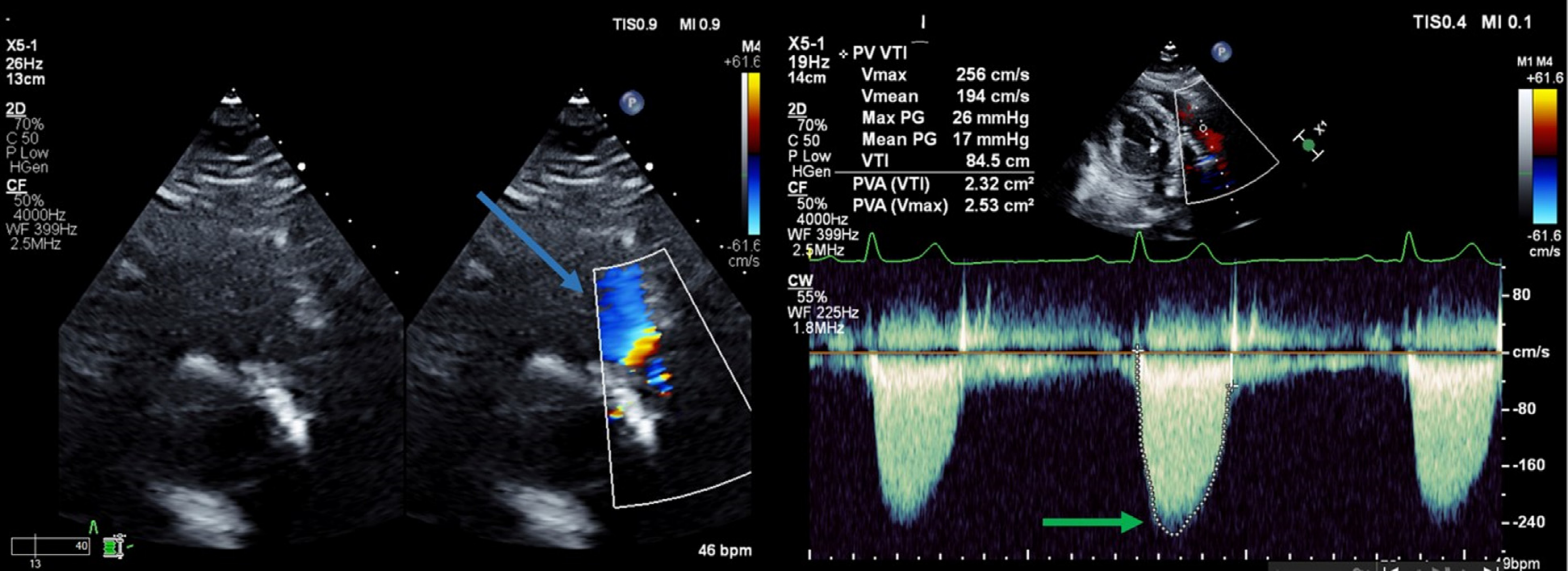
Transthoracic Echocardiogram Imaging uses color Doppler to highlight flow acceleration during systole, marked by the blue arrow. It also features a continuous wave (CW) Doppler analysis of a prosthetic pulmonary valve. The mean gradient across the valve, which is indicated by the green arrow, is measured at 17 mm Hg. CF = color flow; max = maximum; PG = pressure gradient; PV = pulmonary valve; PVA = pulmonary valve area; V = velocity, VTI = velocity time integral; 2D = 2-dimensional.
